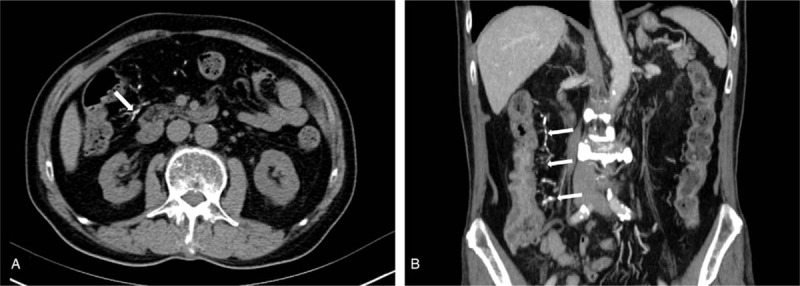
Abdominal CT scan. (A) Non-contrast images showing venous calcification (white arrow) form the ascending colon to the hepatic flexure. (B) Coronal reformatted T images in the venous phase showing wall thickening limited to the ascending colon, and the scope of vein calcification is larger than that of colon wall swelling and thickening.
